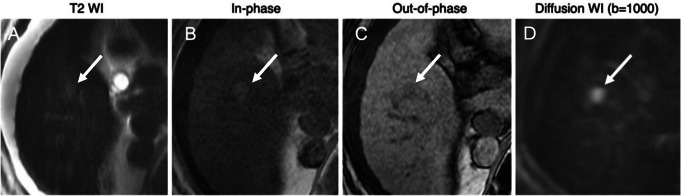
Positive surveillance NC-AMRI. Axial T2-weighted (A) MR image shows a subtle T2 hyperintense mass (arrow) in hepatic segment 5. The mass demonstrates iron sparing on the axial in-phase and out-of-phase T1-weigthed (B, C) MR images. Axial diffusion-weighted (D) MR image shows restricted diffusion within the mass, distinct from background liver parenchyma. All findings are suspicious for HCC and require confirmatory diagnostic testing
